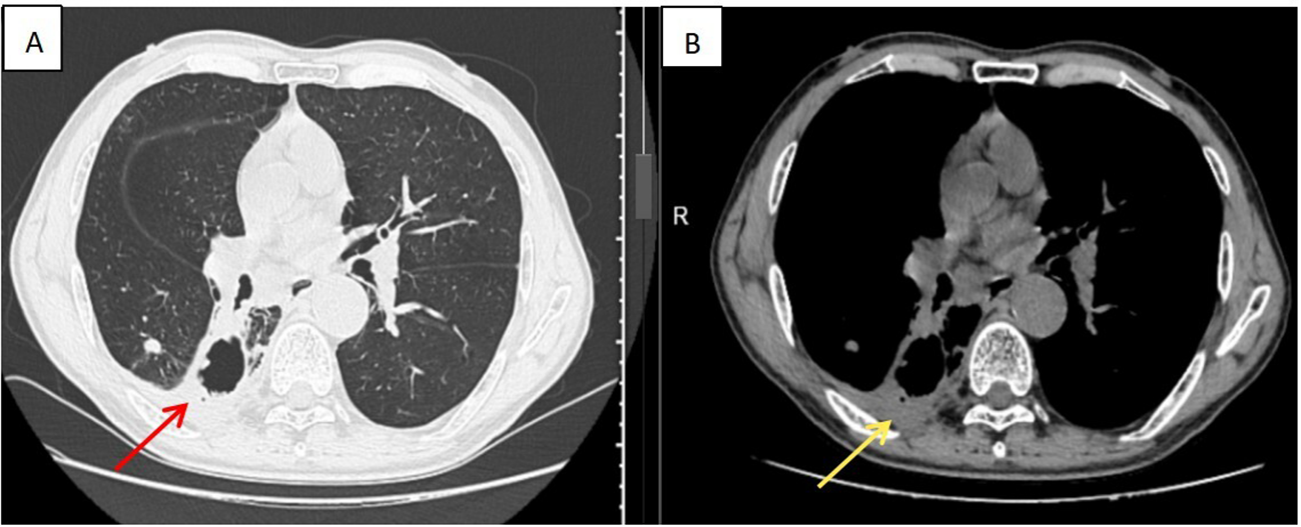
Follow-up chest CT on 6 September 2025 demonstrated significant reduction in the size of the right lung lesion (arrow), measuring 3.2 × 3.1 cm. (A) Red arrow: lung window, (B) yellow arrow: mediastinal window.
